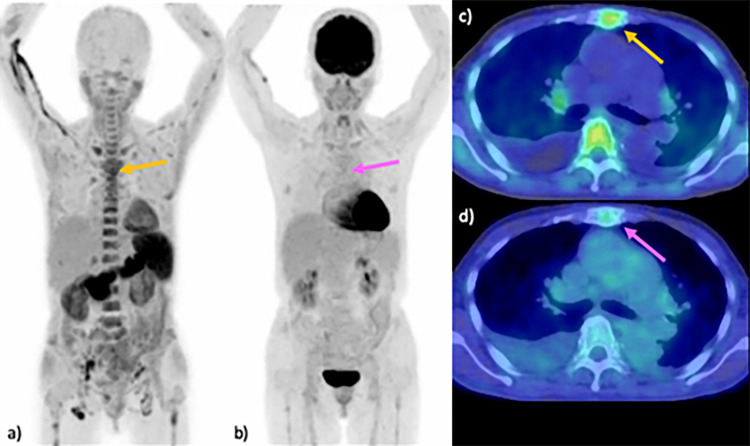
Comparison of PET imaging in POEMS syndrome: 11C-acetate versus 18F-FDG Imaging results of a 69-year-old woman with POEMS syndrome are presented. MIP images from the 11C-acetate PET scan revealed multiple lesions in the vertebrae and sternum (arrow) (a). Lesions in the thoracic vertebrae and sternum are detected using 18F-FDG PET (arrow) (b). An axial fused PET/CT image at the level of the thoracic vertebra demonstrates increased uptake of 11C-acetate in the sternal lesion (SUVmax 6.4) (arrow) (c), in contrast to minimal uptake observed with 18F-FDG (SUVmax 2.0) (arrow) (d). FDG, fluorodeoxyglucose; MIP, maximum intensity projection; SUV, standardized uptake value
